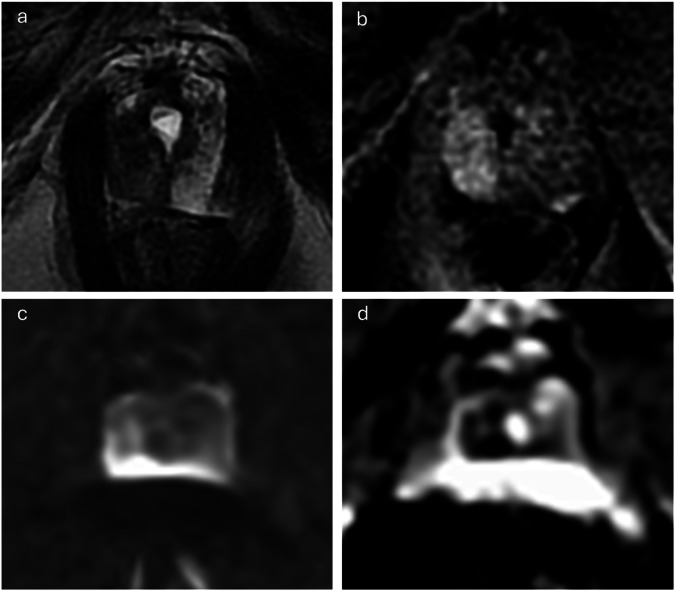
Image quality was classified as inadequate consistently on PI-QUAL v1, v2 bpMRI and v2 mpMRI. Image of a 74-year-old patient scanned at 1.5-T MRI with a presenting PSA of 6.83 ng/mL and a prostate volume of 20 cc (PSA density: 0.34 ng/mL/mL). PI-QUAL v1 assessment reveals sub-optimal image quality for axial T2WI (a), DWI (c), and ADC (d) sequences, while the DCE image (b) is diagnostically acceptable. Consequently, the overall v1 quality is 2/5 (only one sequence is of diagnostic quality), indicating inadequate image quality (1/3) on a 3-point scale. Correspondingly, the overall mpMRI image quality under PI-QUAL v2 is inadequate (1/3), with T2WI and DWI-ADC scoring 0/4 each, and DCE scoring 2/2
